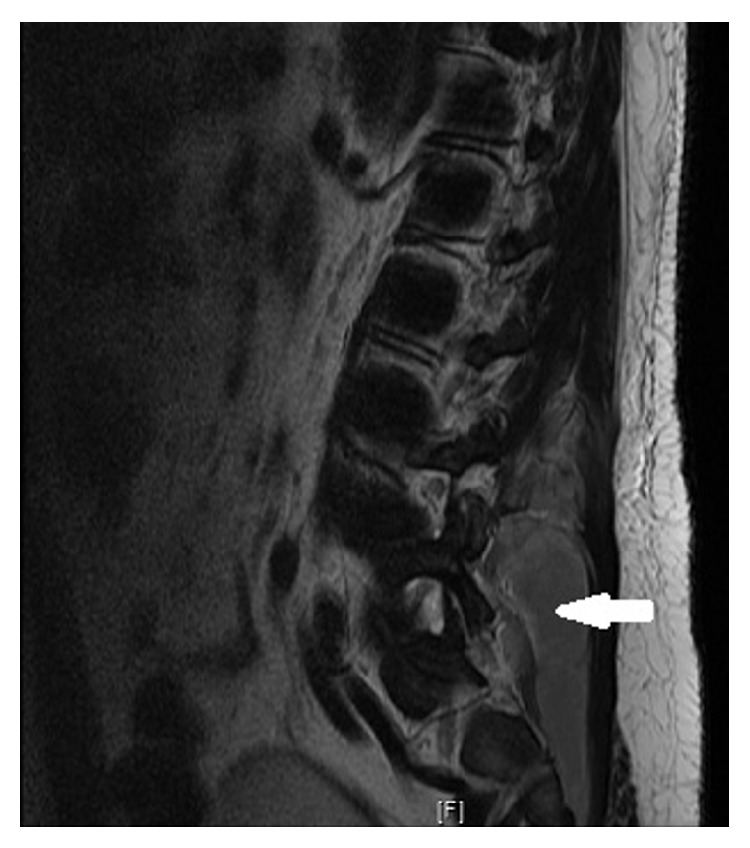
Sagittal view of T2 diffusion MRI lumbar spine showing heterogeneous lesions (arrow) involving the paraspinal muscles with a ring enhancement consistent with abscess.
